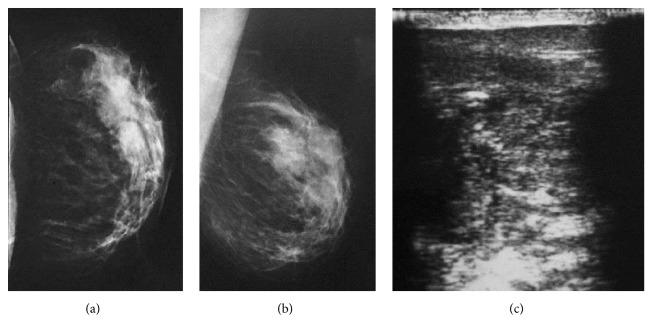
Mammogram images (a and b) showing a mass with irregular posterior limits, which on ultrasound image (c) is a hypoechoic mass with irregular and indistinct margins at the 2 o'clock position of the left breast measuring approximately 4.2 cm in maximal diameter* (Collection of the Department of Radiology, University Teaching Hospital of Lomé)*.
